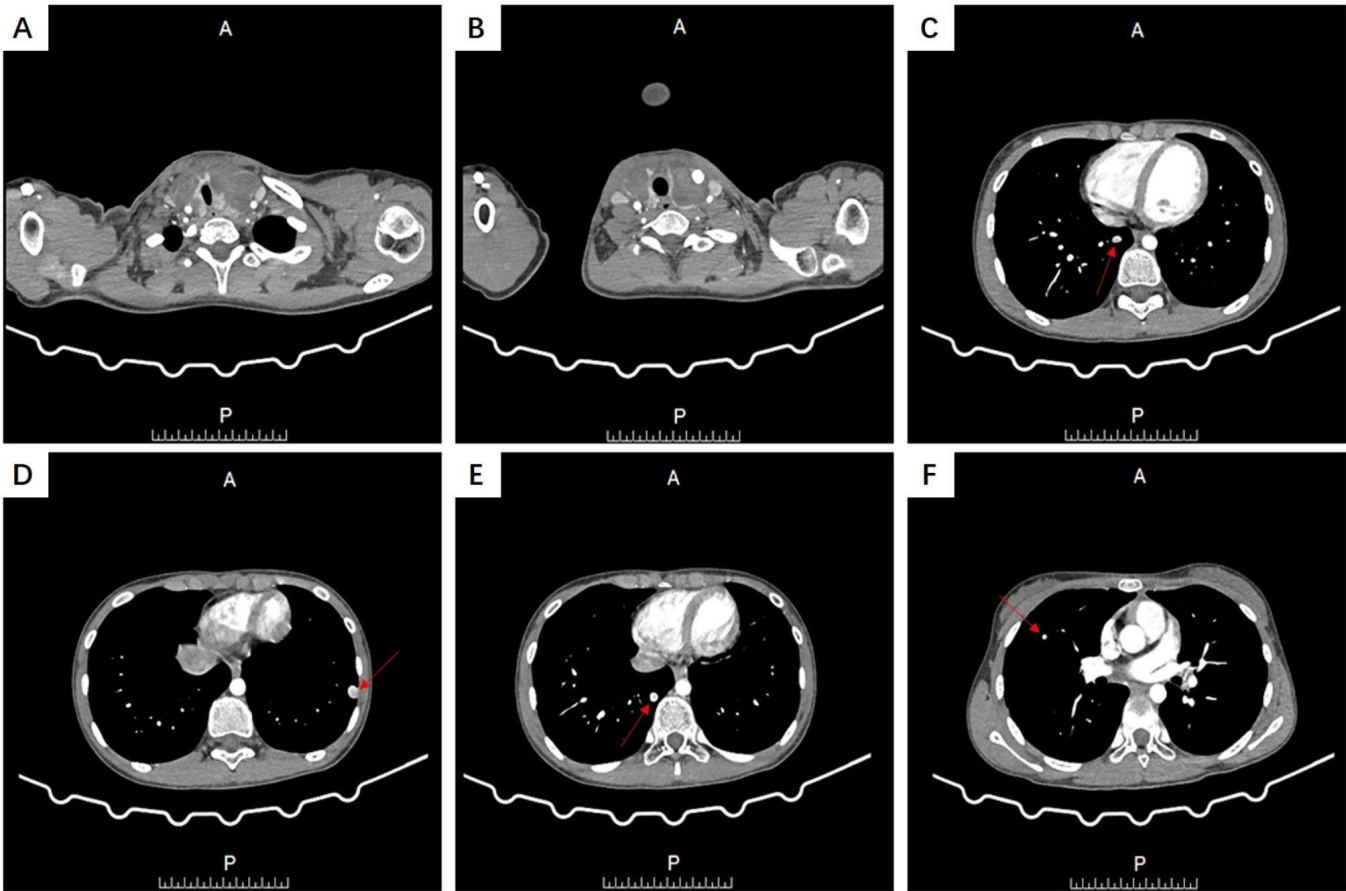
The enhanced computed tomography (CT) scanning showed abnormalities in thyroid and lung. (A-B) Multiple nodules in thyroid. (C-F) Multiple nodules in both lungs
